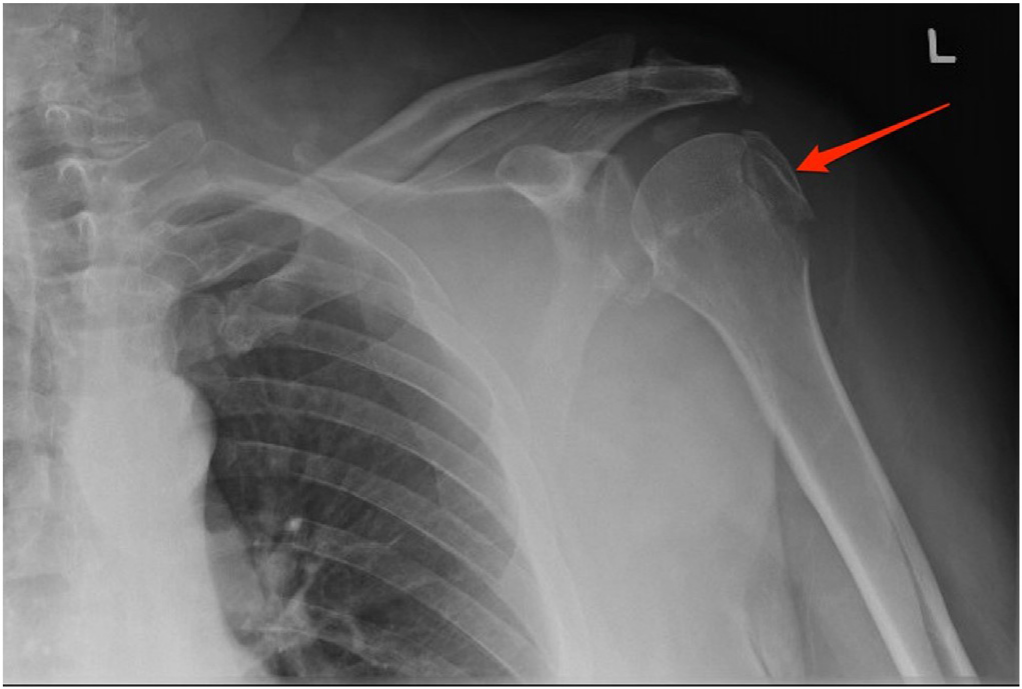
Radiography from initial emergency room visit. *Red arrow* indicates acute greater tuberosity fracture.
